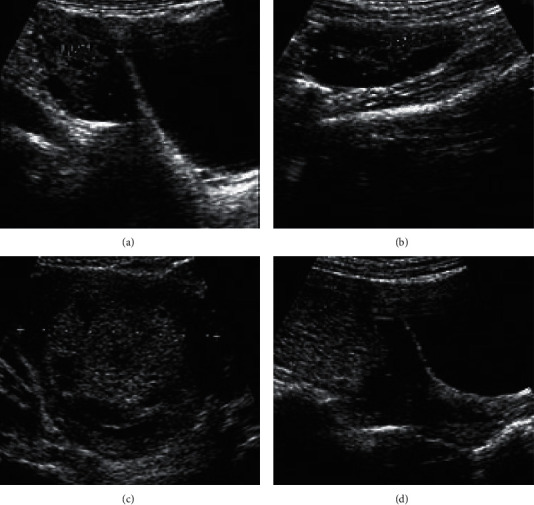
Postoperative ultrasound images of a 37-year-old patient undergoing total hysterectomy. ((a), (b)) Transabdominal; ((c), (d)) transvaginal uterus.
